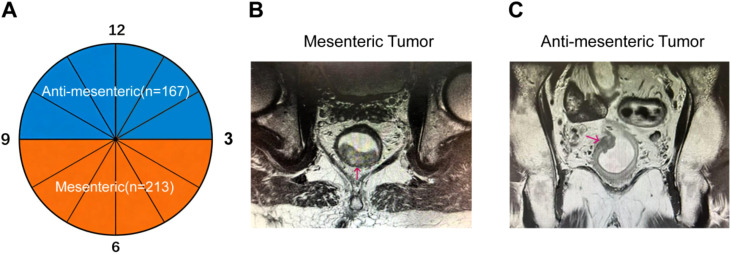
(A) Rectal tumor location in patients placed in the lithotomy position is categorized as follows: mesenteric tumors are situated between the 3 and 9 o’clock positions, while anti-mesenteric tumors are located between the 9 and 3 o’clock positions. Corresponding axial contrast-enhanced MRI images demonstrate the deepest extent of tumor invasion in the mesenteric (B) and anti-mesenteric (C) regions of the rectum.
